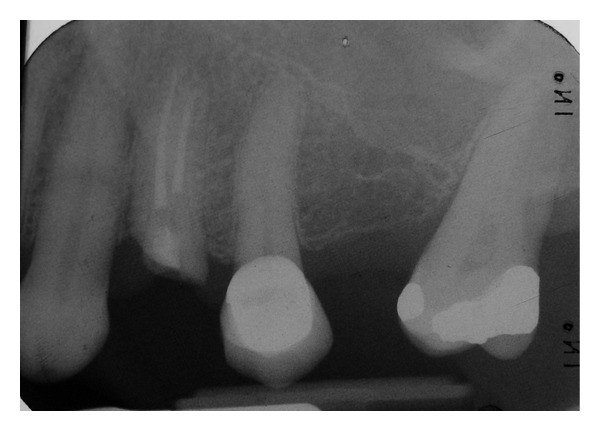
Periapical radiograph of UL4 shows intact root fillings, oblique fracture and a healthy root.
